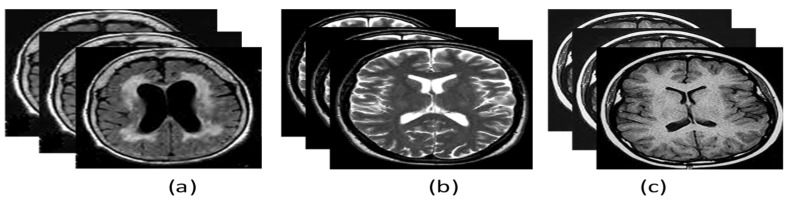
Brain axial MRI (T1 and T2 weighted) data for (a) Alzheimer’s Disease, (b) Mild Cognitive Impairment and (c) Control Normal.
